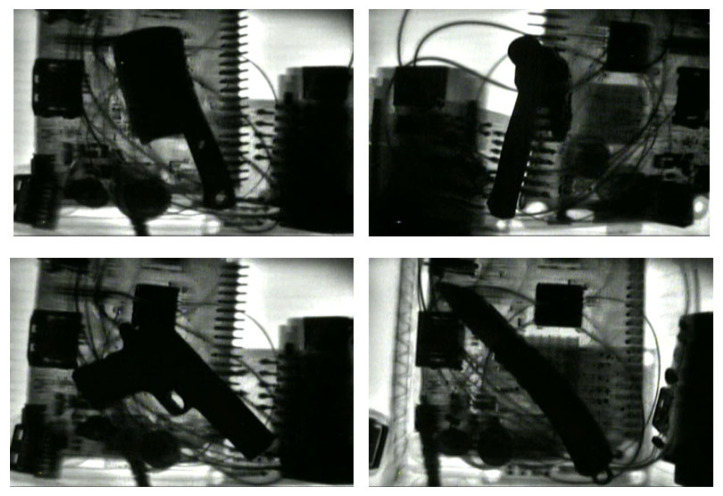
X-ray images of a single prohibited item on a complex background.
